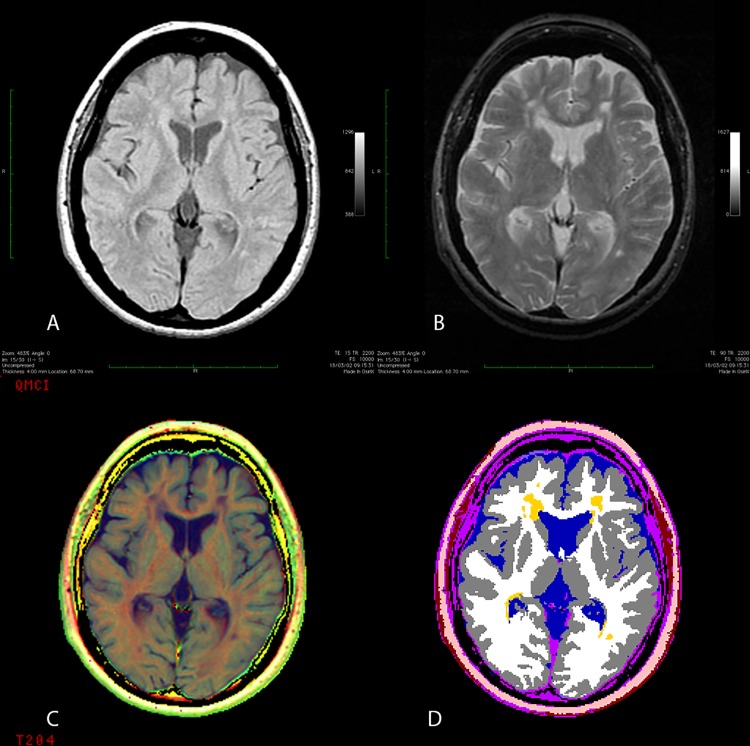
Axial image of a Multiple Sclerosis patient. (A) Proton density-weighted image; (B) T2-weighted image; (C) corresponding multiparametric image; (D) segmented image. In the segmented image, white matter is represented by white, gray matter by gray, cerebrospinal fluid by blue, and lesions by yellow.
